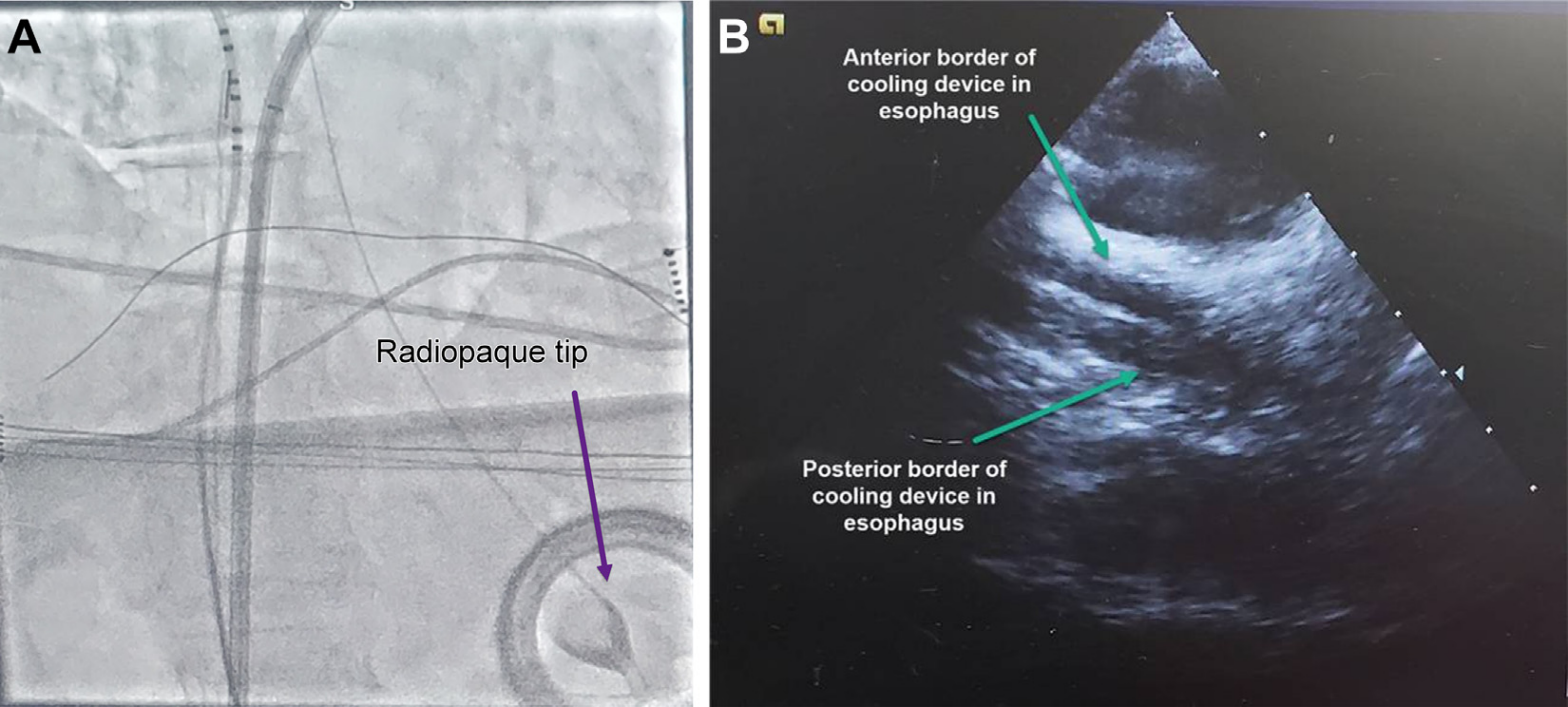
A: Visualization of the proactive esophageal cooling device (ensoETM, Attune Medical, Chicago, IL) on fluoroscopy, showing the radiopaque tip below the diaphragm. B: Visualization of the proactive esophageal cooling device (ensoETM, Attune Medical) on intracardiac echocardiography, showing anterior and posterior borders of the device in the esophagus.
